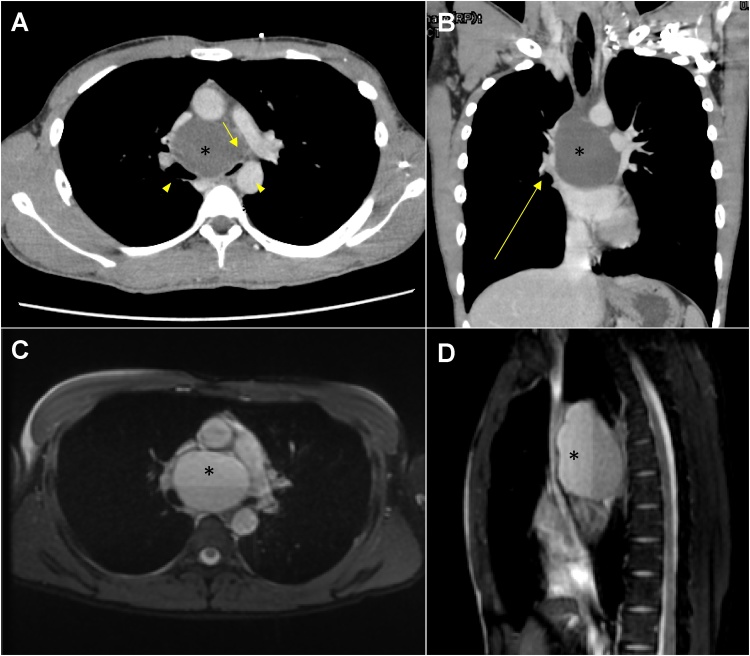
Preoperative images. A, B. Chest computed tomography. A mediastinal mass was noted in the subcarinal space (*). Compression of the right pulmonary artery (short arrow) and airway (arrowhead). Delayed enhancement of the right pulmonary vein and airway was seen at the tracheal bifurcation (long arrow). (A: Axial, B: Coronal). C, D. Fat-suppressed T2-weighted magnetic resonance imaging. A two-layered simple cyst (*) was observed, which indicated infection or bleeding inside the cyst. (A: Axial, B: Sagittal).
